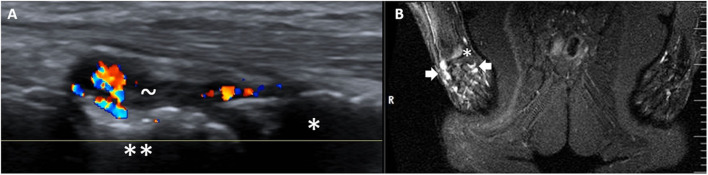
Inflammatory activity in the right (R) wrist as shown by ultrasound (A) and WBMRI (STIR) (B). * = radius,  = schaphoid bone, ~ = synovial hypertrophy with Doppler activity, white thick arrows = high signal intensity in the wrist compatible with inflammation.
